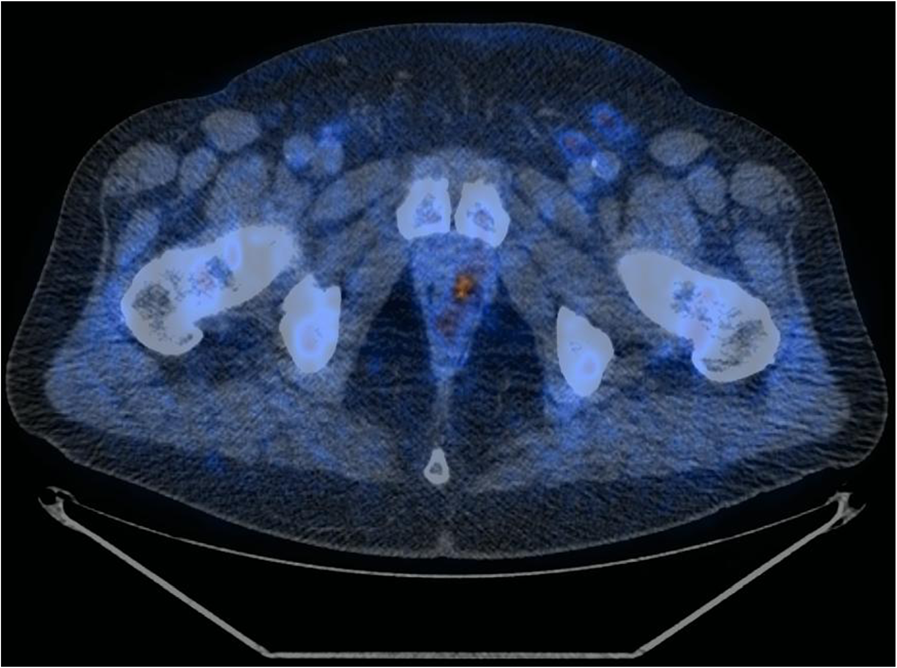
True positive case (PET/CT axial scan). This scan shows a focal uptake of 11C–choline localized on the left paramedian versant of the PB
